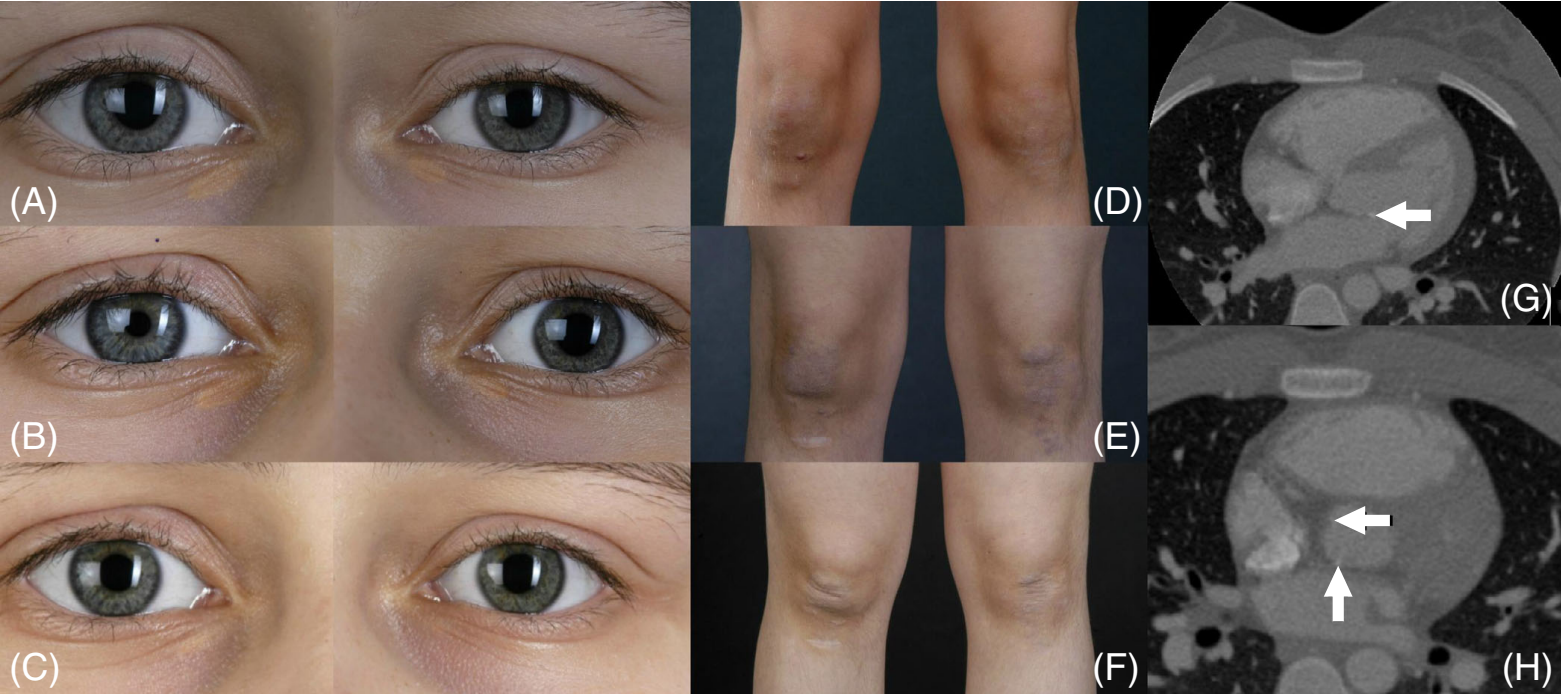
Clinical pictures and CT scan of patient 1. Xanthelasma at age 11.2 (A), 11.9 (B), and 13.3 years (C). Xanthomata at age 8.8 (D), 11.2 (E), and 13.3 years (F). CT scan with cardiac calcifications at age 13.5, arrows indicate site of calcifications (G, H). CT, computed tomography
